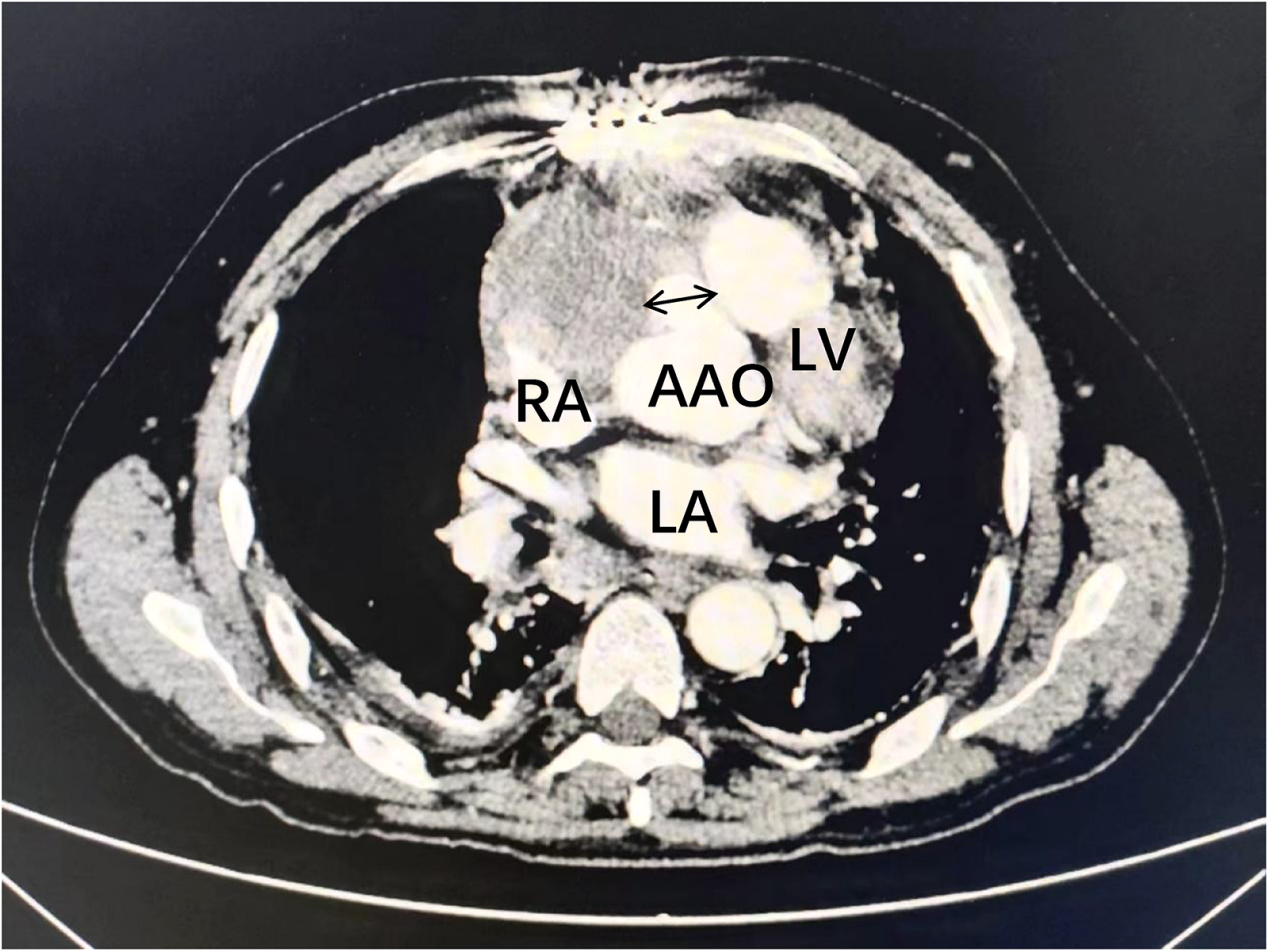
Computed tomography angiography (CTA) suggests localized nodular protrusions in the right coronary sinus, measuring approximately 1.4 × 1.2 cm (black arrow). LA, left atrium; LV, left ventricle; RA, right atrium; AAO, ascending aorta.
